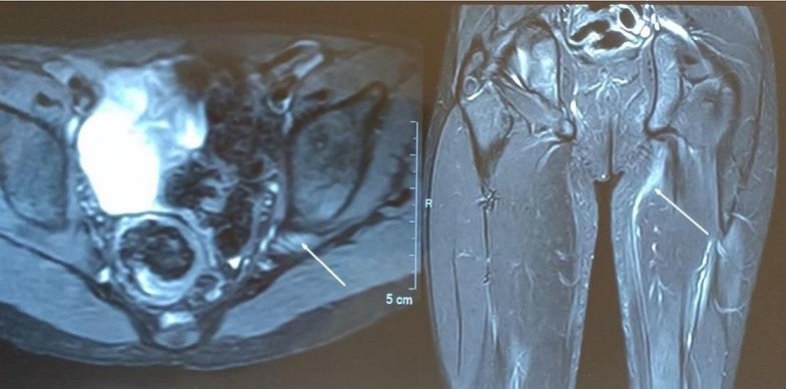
magnetic resonance imaging locating the peripheral nerve lesion
